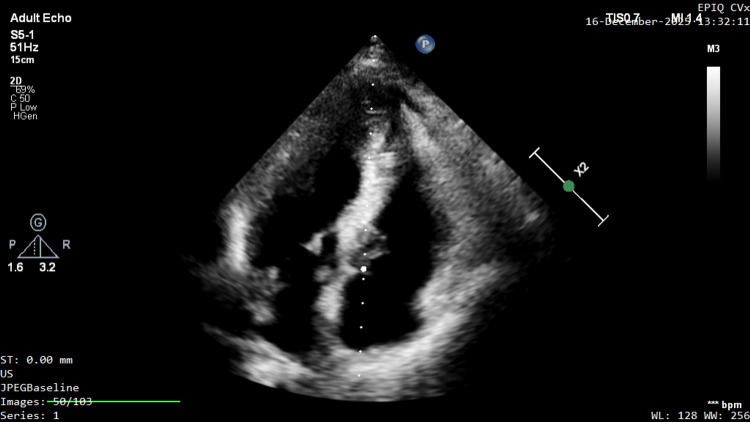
Standard apical four-chamber view in a patient followed for apical HCM, demonstrating marked apical thickening (2.0 cm). Note that the apical aneurysm is not visible in this standard imaging plane.
